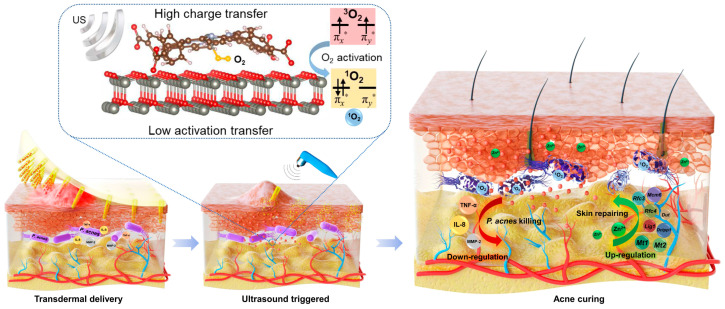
Sonocatalytic acne treatment via efficient ultrasound ion therapy with MN patch. Reproduced with permission [135] American Chemical Society.
